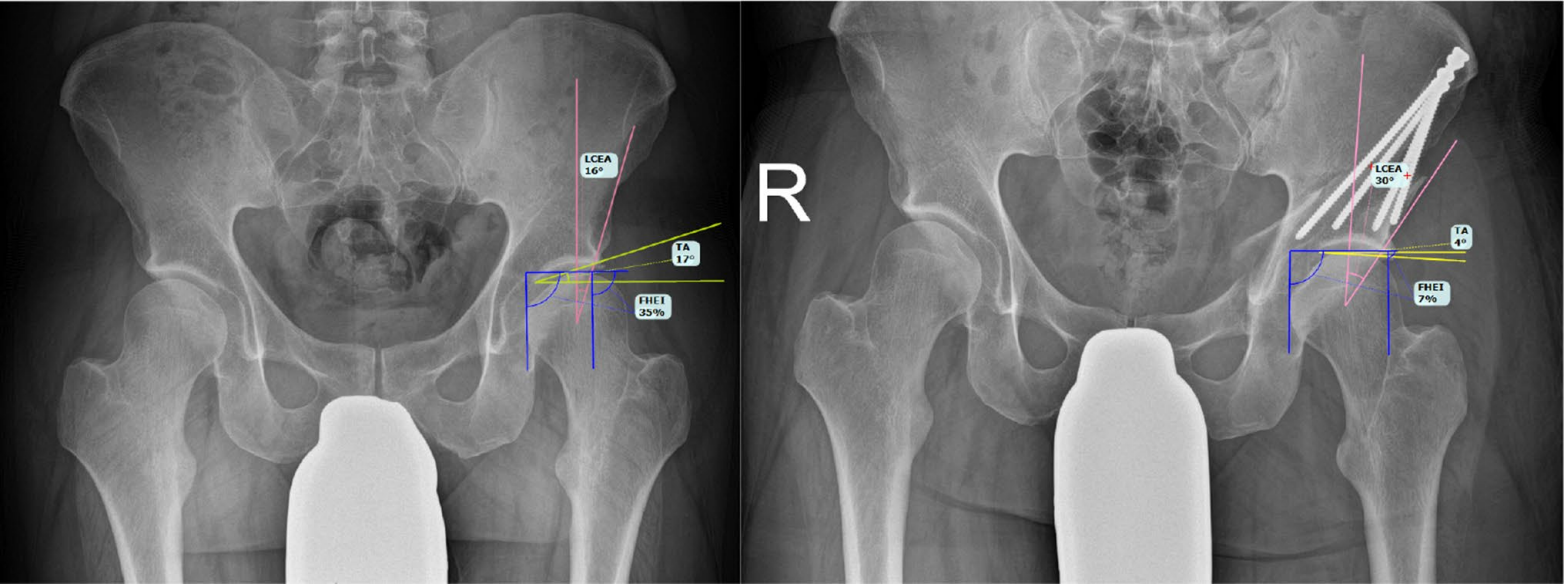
Radiological measurement of lateral center edge angle (LCEA), Tönnis angle (TA) and femoral head extrusion index (FHEI) on a preoperative and b postoperative a.p. pelvis X-rays
